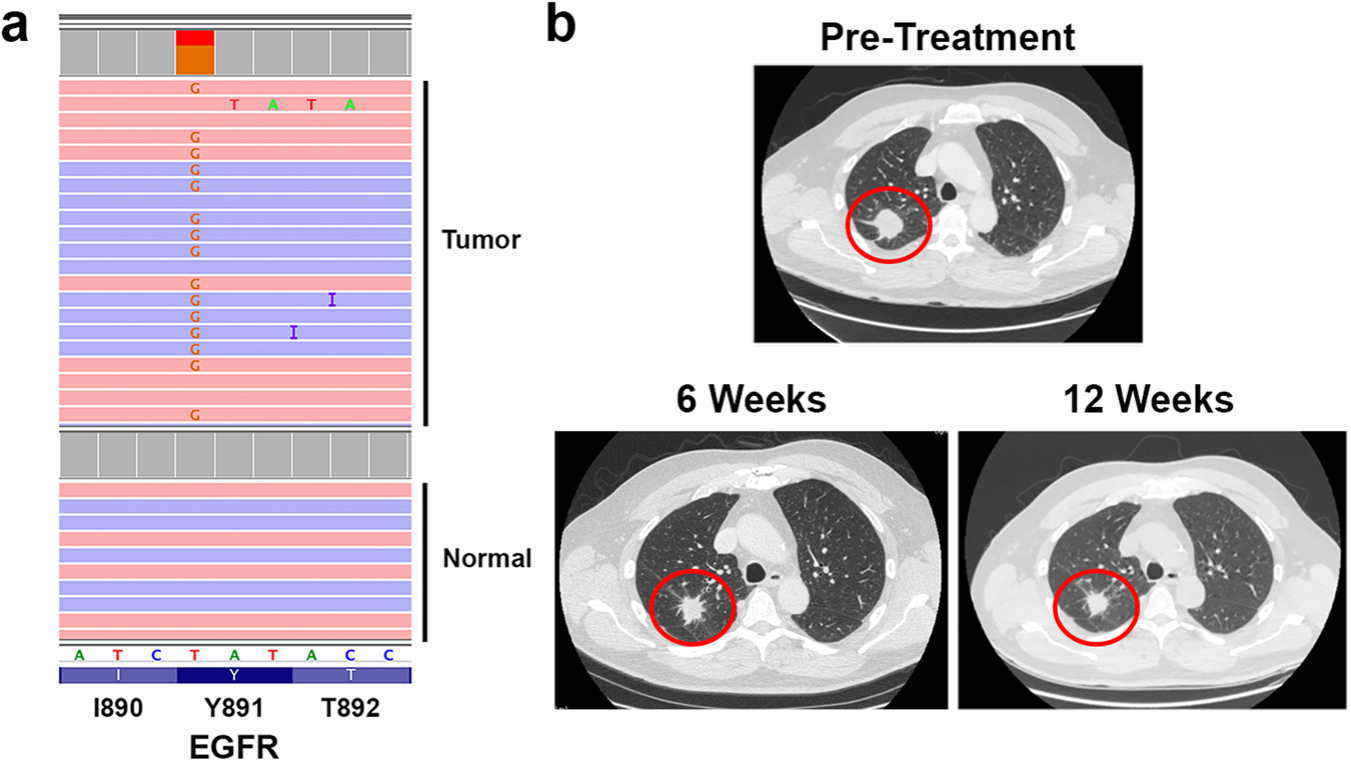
Identification of EGFR Y891D in a patient with advanced NSCLC with EGFR L858R. a Tumor molecular profiling identified EGFR Y891D from a resected brain metastasis prior to initiation of systemic therapy. Pink and blue bars represent forward and reverse sequencing reads from tumor and normal germline control. Data are displayed using the Integrative Genomics Viewer. b Computed tomography (CT) imaging of the chest demonstrating the primary lung mass prior to treatment and after 6 weeks and 12 weeks of osimertinib therapy. After 6 weeks of osimertinib, the tumor decreased in size from 3.2 to 2.6 cm (−18.75%), consistent with stable disease by RECIST criteria. There was no further change in size after an additional 6 weeks of treatment.
